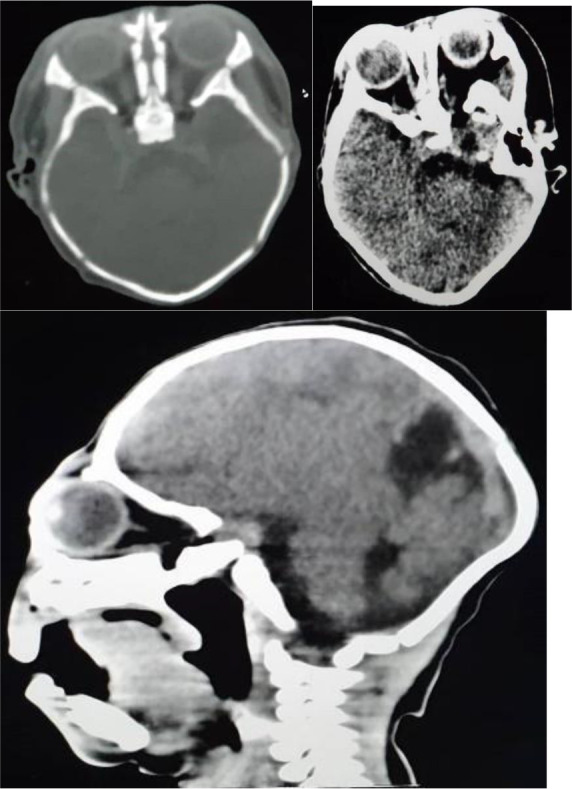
Head CT scan showing posteriorly situated mal-developed mono-ventricle communicating with a dorsal cyst, absent falx, inter-hemispheric fissure and corpus callosum and fusion of the thalami.
